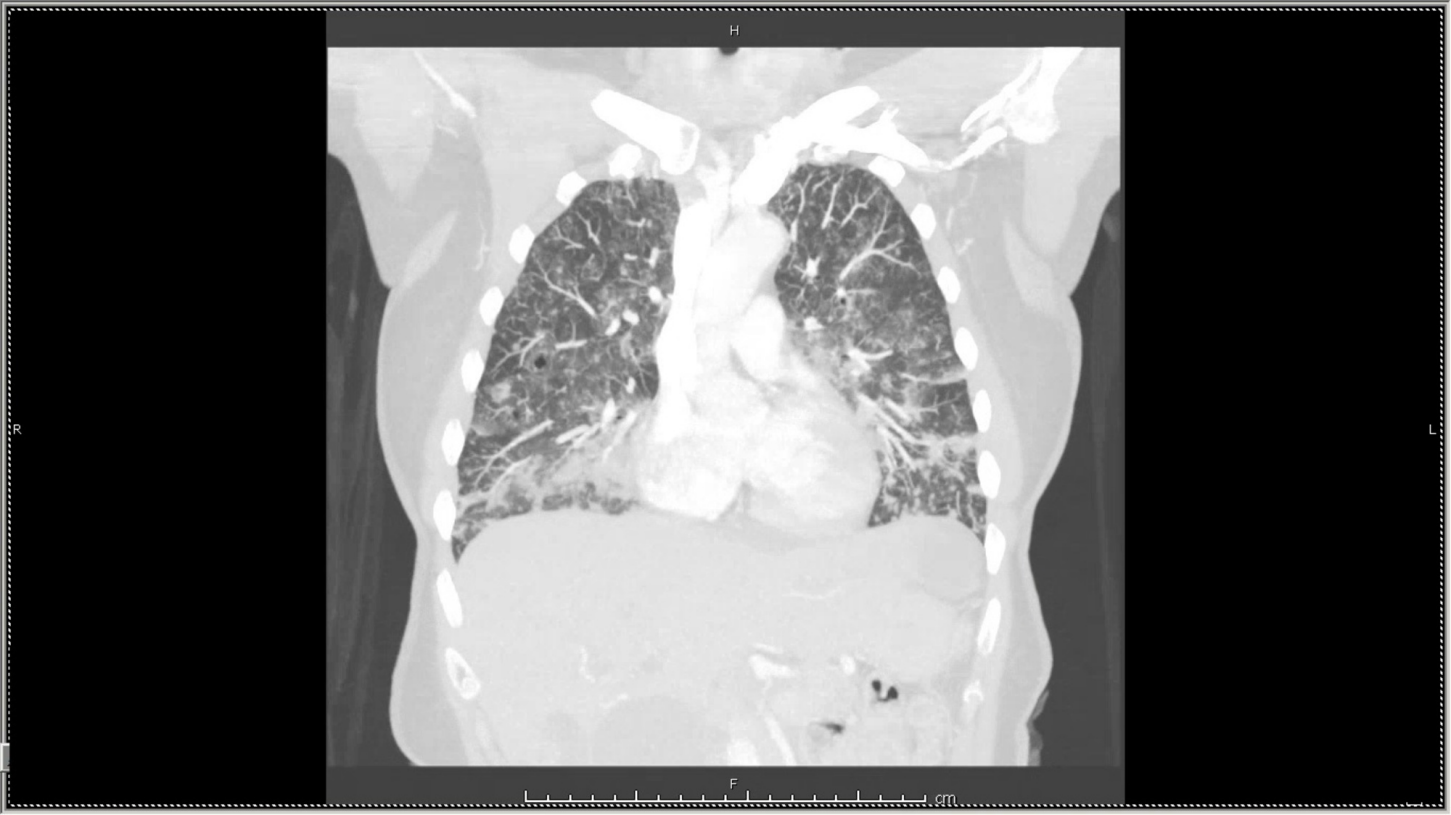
Chest Computed Tomography Scan Cheerios sign visible in lung tissue.
